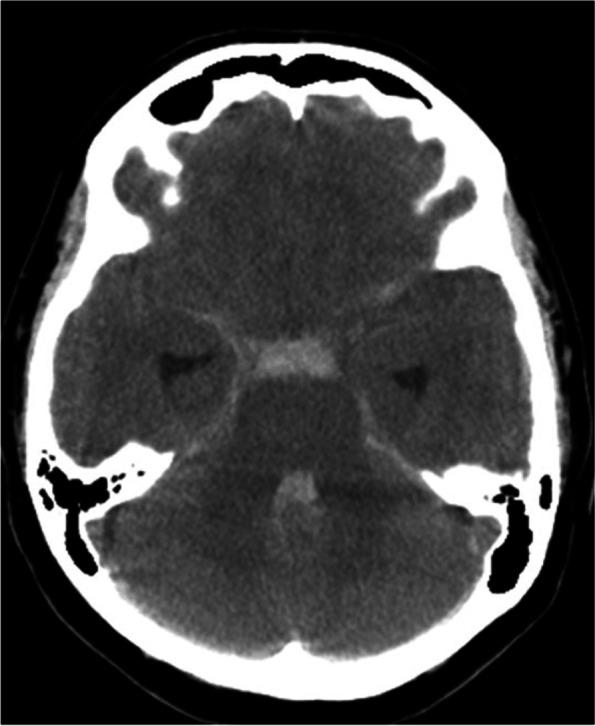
Computed Tomography scan of the patient at admission. Computed Tomography showing subarachnoid hemorrhage
